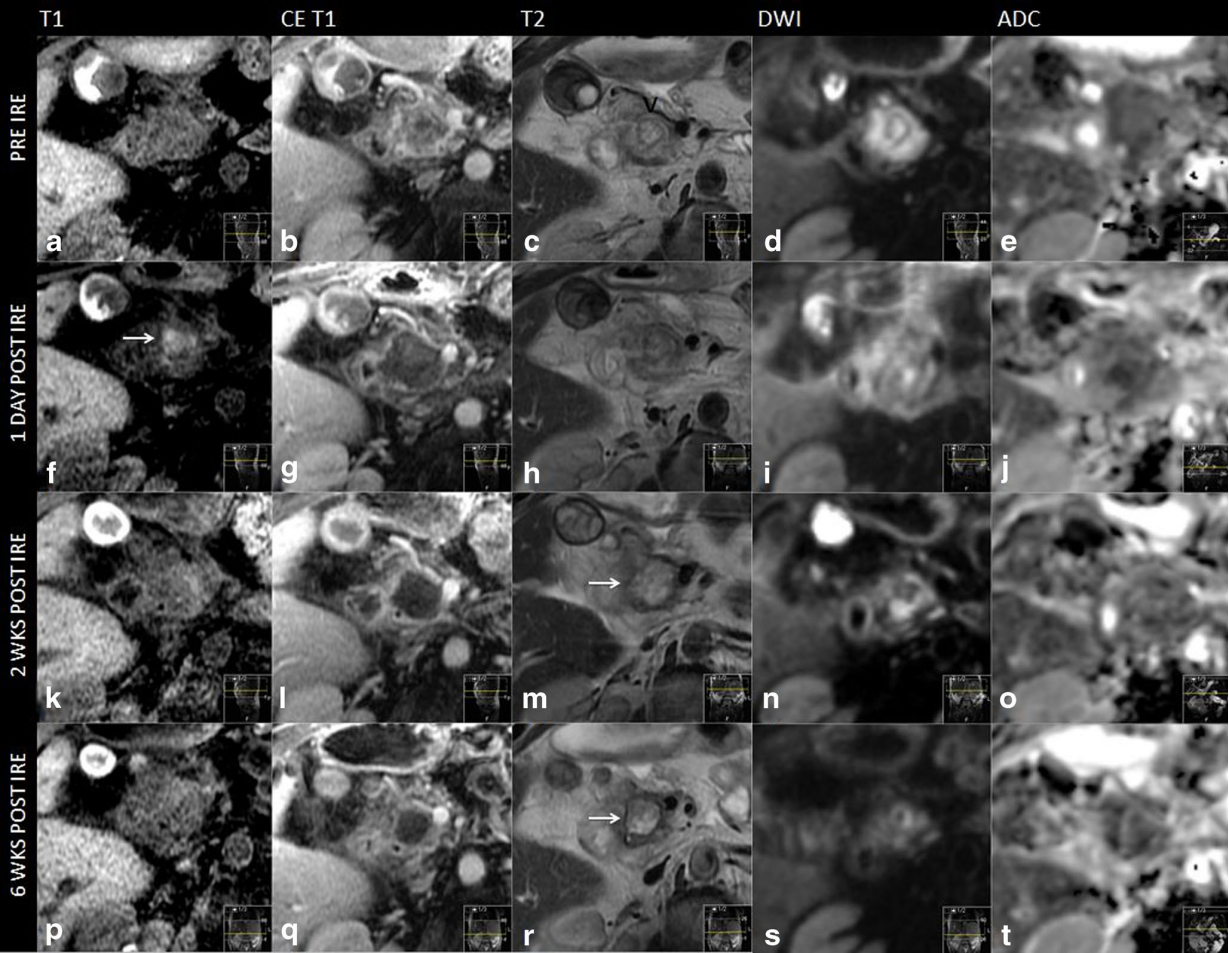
Prior to IRE:* (a) Isointense tumour on T1 sequence (b) Hypointense (-) tumour on T1 sequence (portal venous phase) (c) Hyperintense tumour on T2 sequence (d) Hyperintense (++) tumour on DWI-b800 sequence (e) Hypointense (-) tumour on ADC map. *1 day post-IRE:* (f) Isointense IRE ablation zone with small hyperintense blood residues on T1 sequence (g) Hypointense (---) IRE ablation zone plus rim-enhancement surrounding the treated area on T1 sequence (portal venous phase) (h) Hyperintense (+) IRE ablation zone on T2 sequence (i) Hyperintense (+) IRE ablation zone on DWI-b800 sequence (j) Isointense IRE ablation zone on ADC map. *2 weeks post-IRE:* (k) Isointense IRE ablation zone on T1 sequence (l) Hypointense (---) IRE ablation zone plus rim-enhancement surrounding the treated area on T1 sequence (portal venous phase) (m) Hyperintense (+) IRE ablation zone plus hypointense rim enhancement surrounding the treated area on T2 sequence (n) Hyperintense (+) IRE ablation zone on DWI-b800 sequence (o) Isointense IRE ablation zone on ADC map. *6 weeks post-IRE:* (p) Isointense IRE ablation zone on T1 sequence (q) Hypointense (--) IRE ablation zone on T1 sequence (portal venous phase) (r) Hyperintense (+) IRE ablation zone plus hypointense rim enhancement surrounding the treated area on T2 sequence (s) Hyperintense (+) IRE ablation zone on DWI-b800 (t) Isointense IRE ablation zone on ADC map
